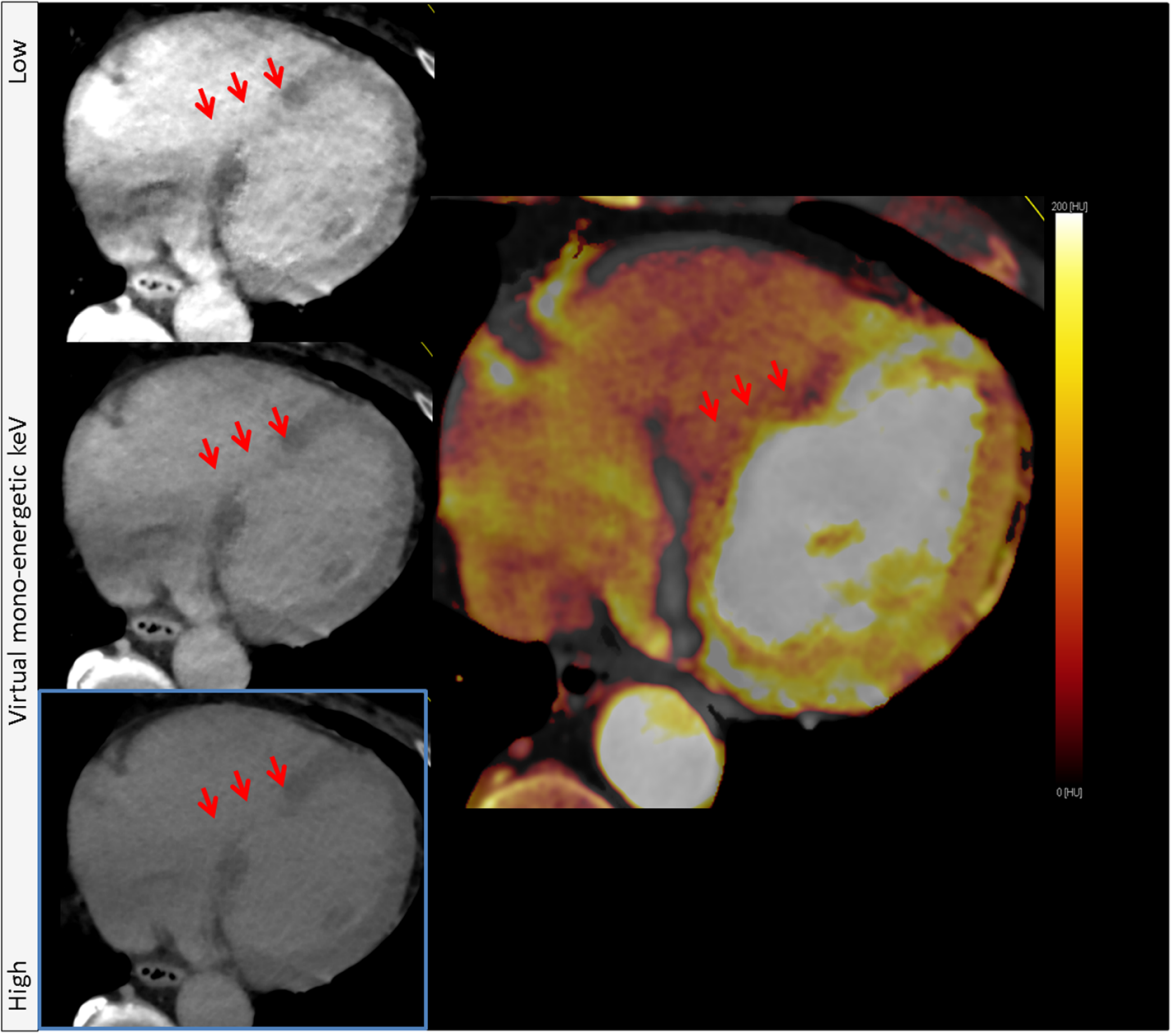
The left images show different level kV LIE-CT images ordered from low to high kV, with the image in the blue box representing the traditional SECT LIE-CT acquisition. The right image represents the iodine map. The red arrows indicate hyper-enhancement of the infarcted areas of the myocardium
